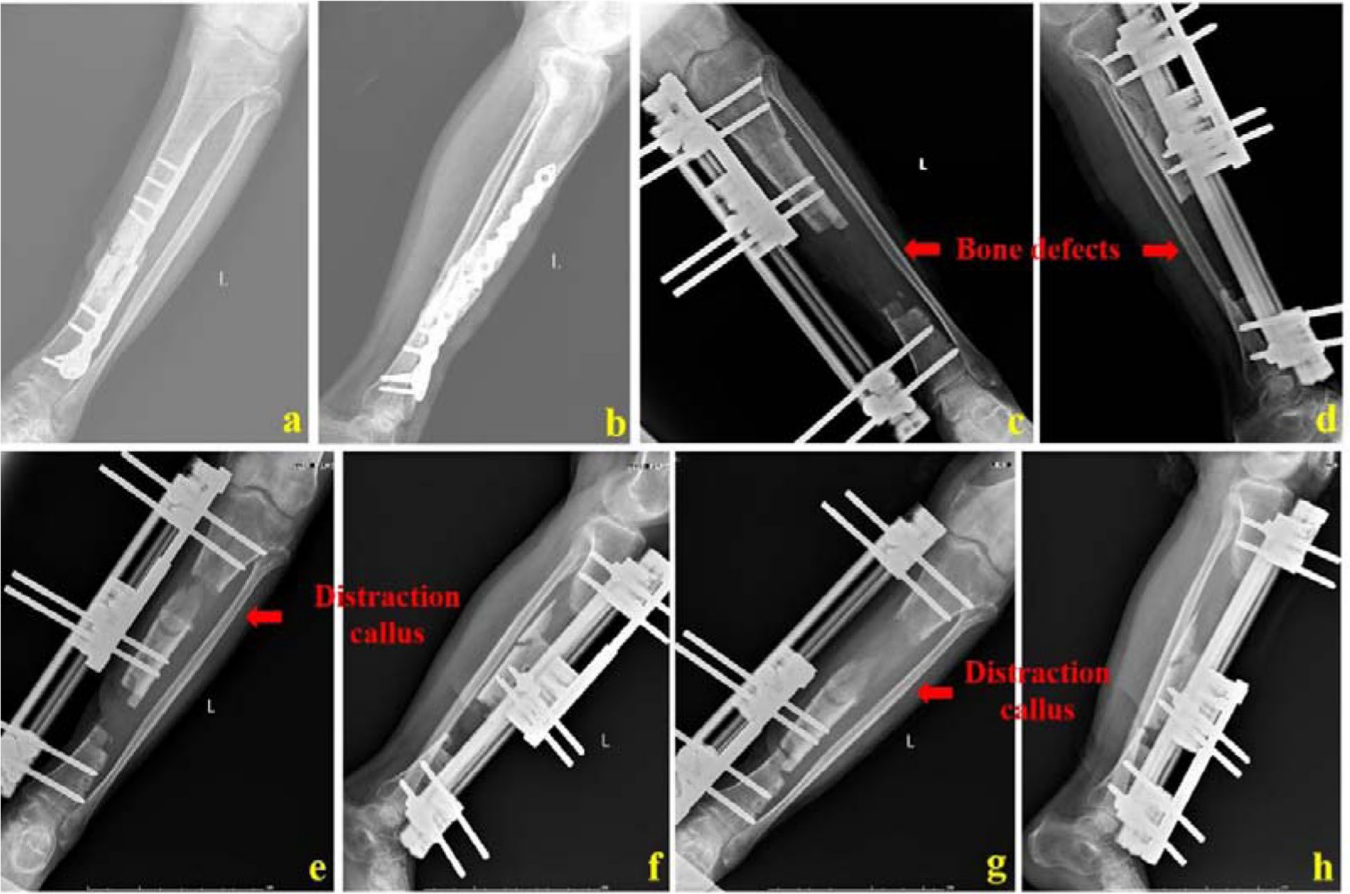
A 55-year-old male was successfully treated by bone transport combined with bone graft and internal fixation technique. a and b: The patient had infectious nonunion of tibia and the plate was broken; c and d: After the whole segment of sequestrum was cut, the bone defect length was 12 cm. At the initial stage, surgeons used a single-arm transport frame for bone transport; e and f: X-ray images when bone transport was performed for one and a half months; g and h: X-ray images when bone transport was performed for 3 months
